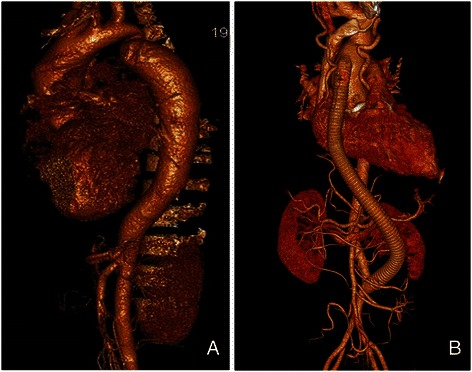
Preoperative thoracic and abdominal computed tomographic angiography (CTA) showing a descending atypical coarctation (a). Postoperative thoracic and abdominal CTA showing the excellent fluent flow of the aorta-lower abdomiml aorta bypass graft (b)
